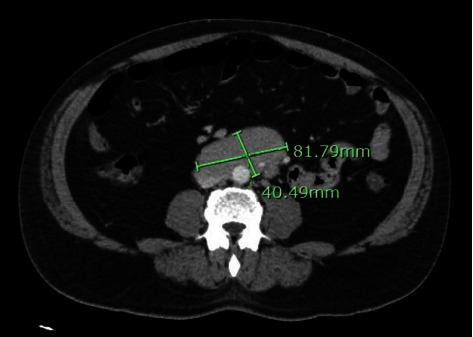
CT Abdomen and Pelvis with contrast showing enlarged preaortic lymph node (measured as 8 × 4 cm on axial view).
